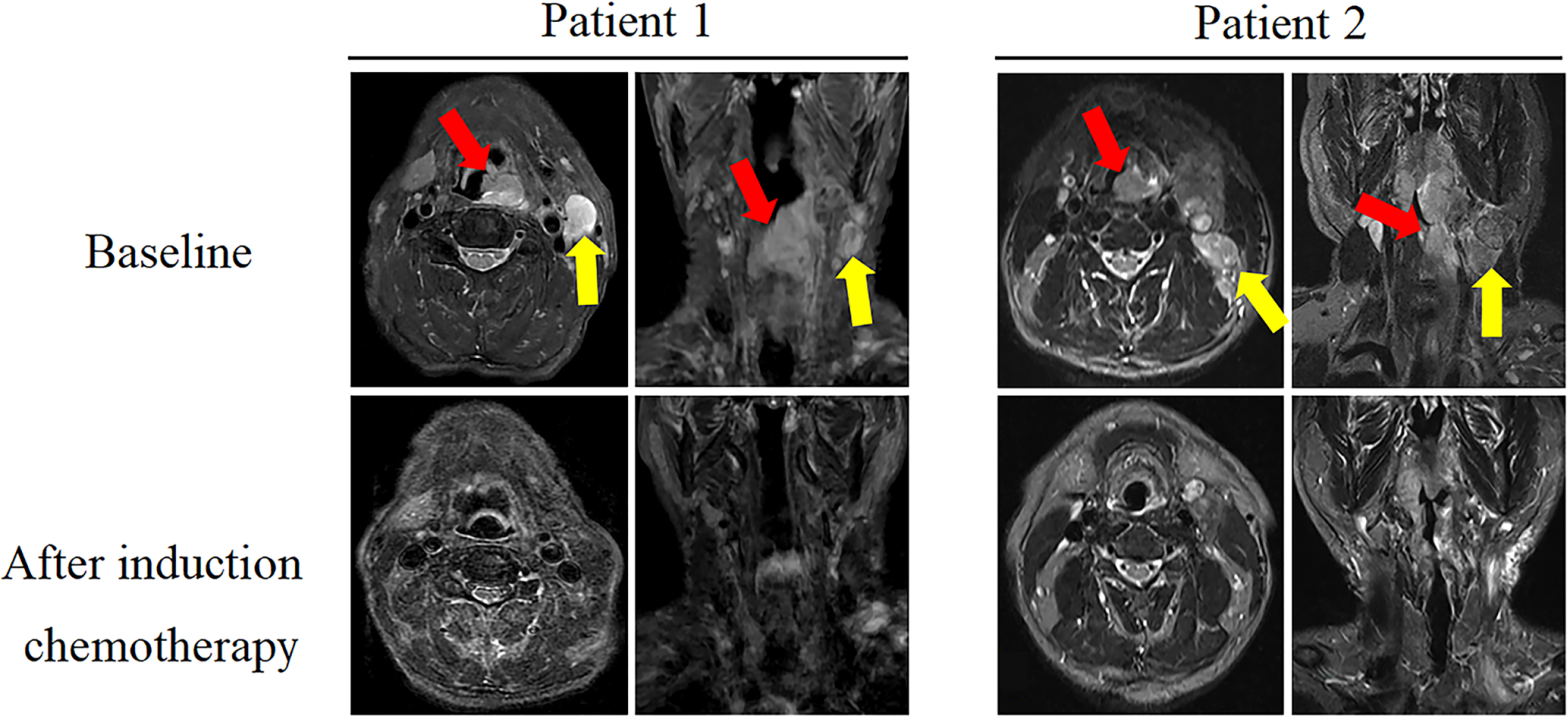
Comparison of magnetic resonance imaging (MRI) images (axial and coronal T2WI images) between baseline and after two cycles of induction chemotherapy in two typical cases. In the baseline image, red and yellow arrows indicate primary lesion and lymph node metastasis, respectively. After induction therapy, the corresponding area of the lesion disappeared.
